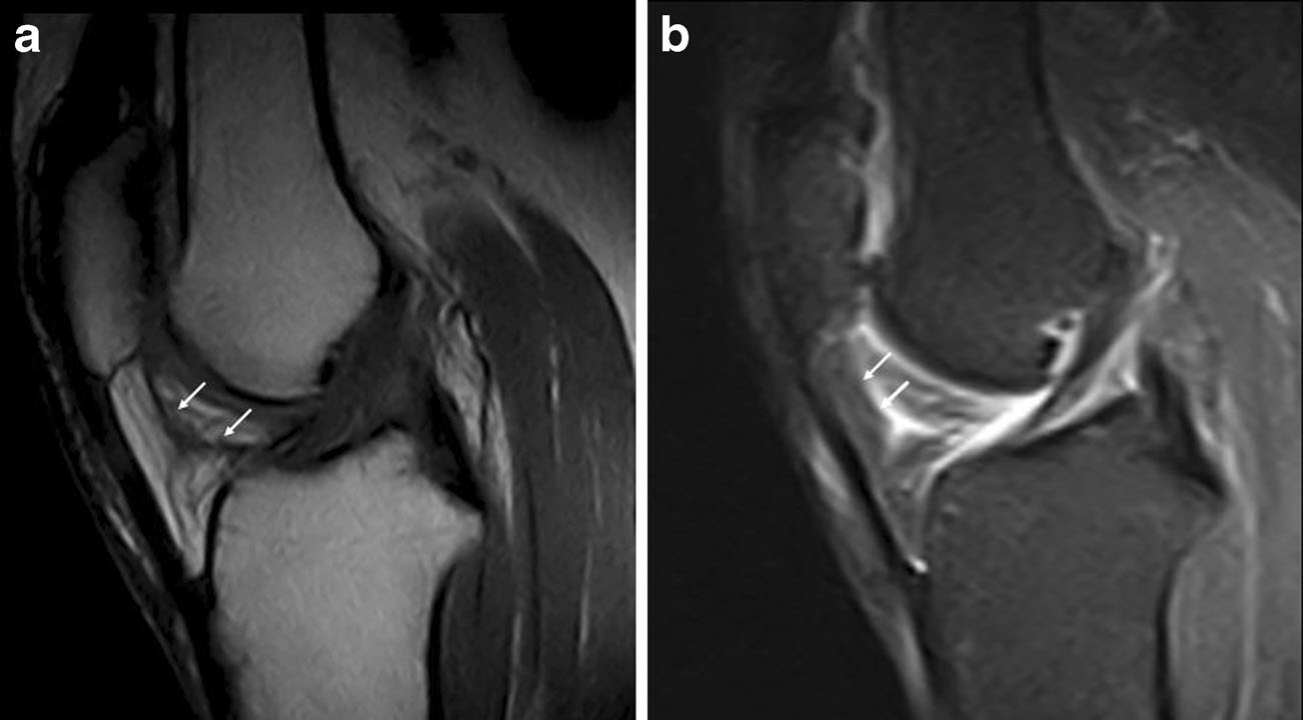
Infrapatellar thickened plica. Sagittal T1w (a) and proton density with fat saturation (b) MR images show thickened infrapatellar plica (*arrows*) with associated oedema of HFP
